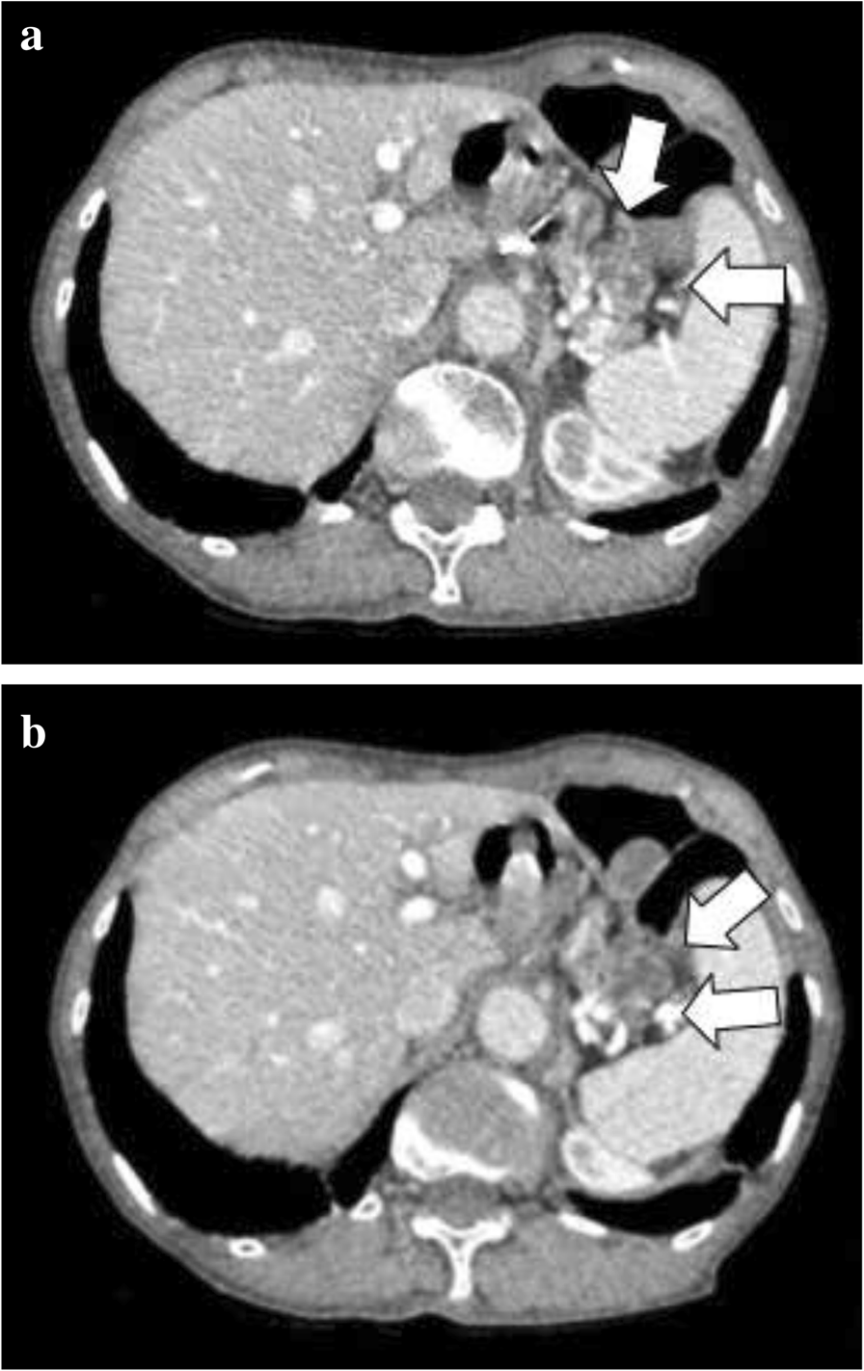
Pre-operative computed tomography findings. Pre-operative computed tomography imaging detected a an adherent mass at the pancreatic tail and b a tumour above the mass
